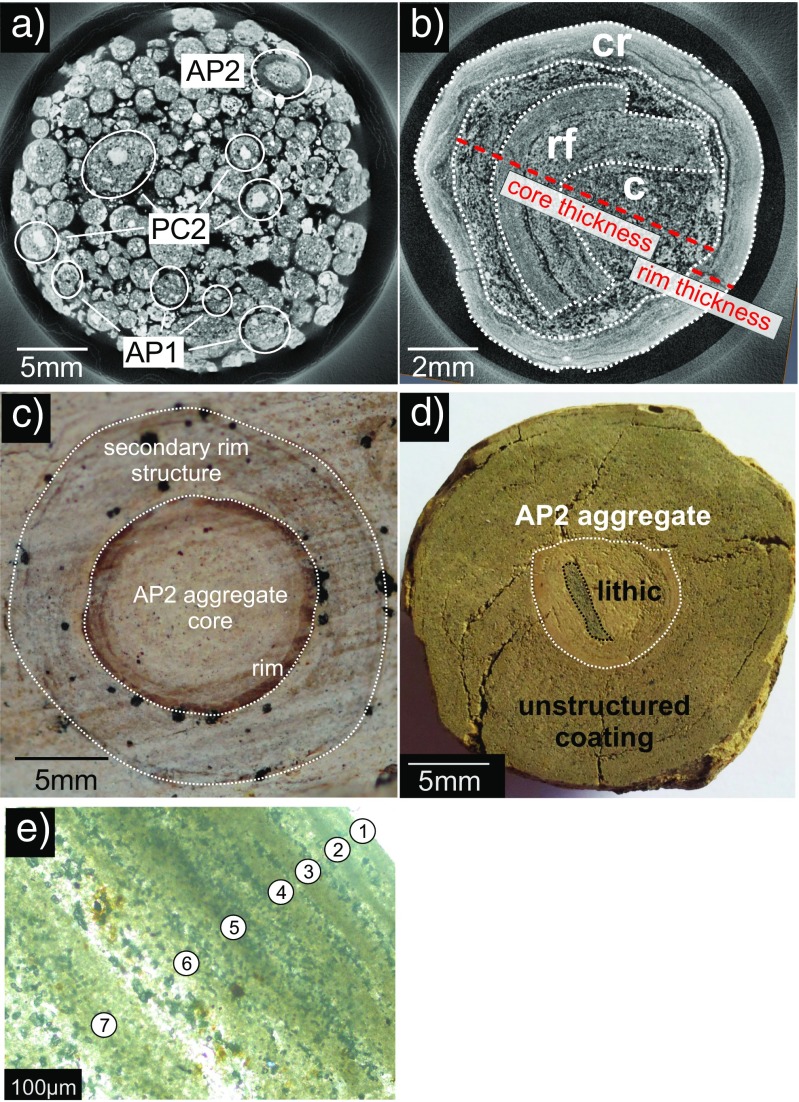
Details of various samples at various resolutions. a X-ray CT analysis of a 2.5-cm-wide drill core from the Secche di Lazzaro succession on Stromboli. The drill core contains PC1, PC2, and AP2 type aggregates. Most aggregates represent the PC1 type. b AP2 similar type aggregate from Empiglione, Italy, 10 mm across. The X-ray image shows a broken rim fragment (rf) being recycled to act as a core (c) of a new accretionary pellet coated by a concentric rim structure (cr). c sample from Caldera del Rey, Tenerife, showing a “double” accretionary pellet: a small, inner accretionary pellet acts as a core for an even larger accretionary pellet. Concentric structures typical for APs are existent in both the inner and the outer rim structures. The whole structure is 20 mm across. The dotted lines indicate the physical and optical separation of the inner accretionary lapilli from the outer rim. d Accretionary pellet sample from Masaya Caldera, Nicaragua. The inner, spherical accretionary pellet (AP2) has a black lithic fragment acting as a core (“armored pellet”). Compared to the Caldera del Rey sample (Fig. 2c), there are no concentric rim features in the outer part of the sample existing. e Close-up of the rim of an AP2 sample of Valle Lungherina, exhibiting the repetitive formation of rims with a grain size fining outwards
